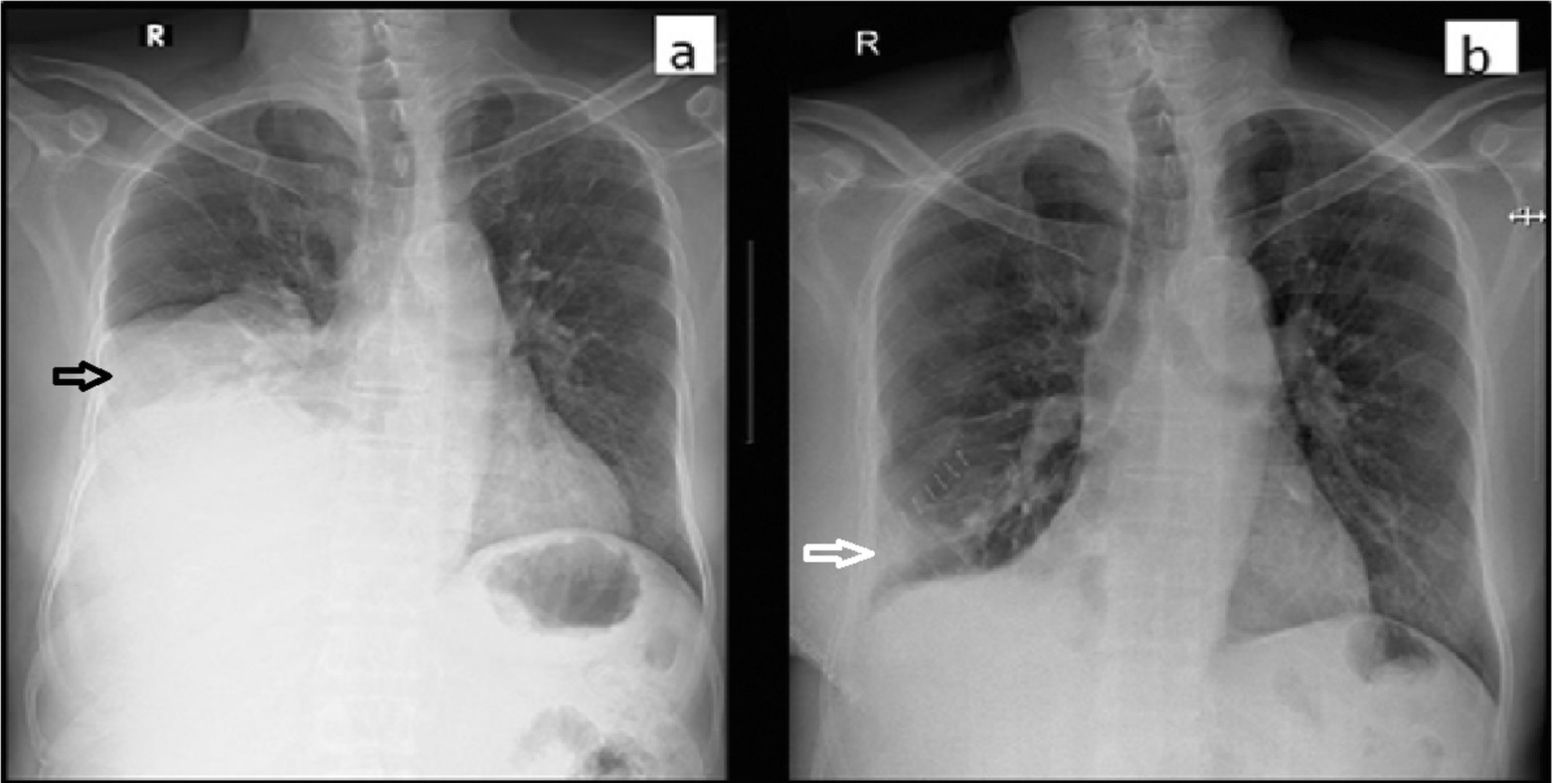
Showing the comparison of chest radiography (a) chest X‐ray before the surgery showing right side middle and lower homogenous opacity in right hemithorax. (b) chest X‐ray after tumour debulking.
